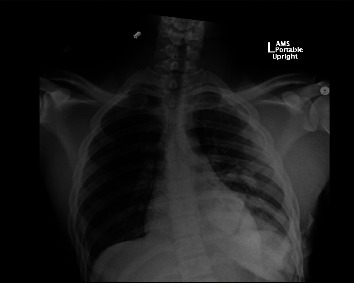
Chest x-ray obtained during the emergency department visit 2. No acute cardiopulmonary process found.
